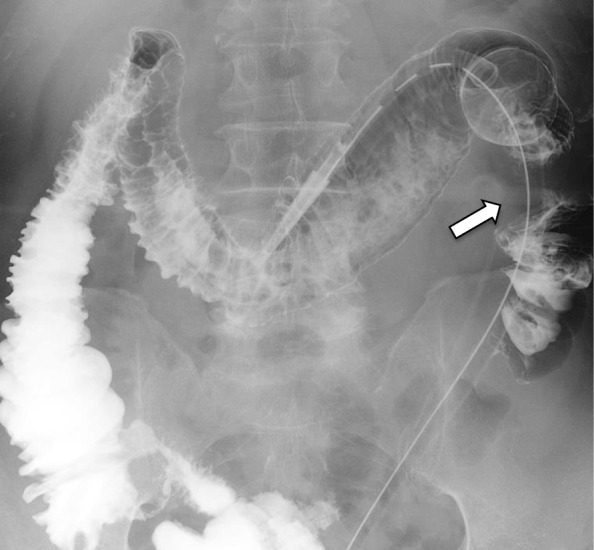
Enema X-ray before operation. Enema X-ray showed light narrowing and a rough mucosal surface in the transverse colon. The arrow shows transverse colon cancer
